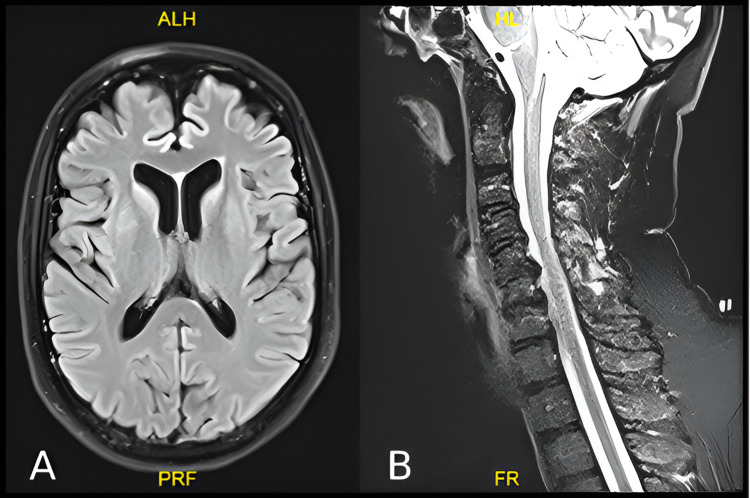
(A) Non-contrast MRI of the brain shows mild age-matched brain involutional changes with no evidence of acute vascular insult. (B) Non-contrast MRI cervical shows multilevel disc lesions with mild neural compression.
